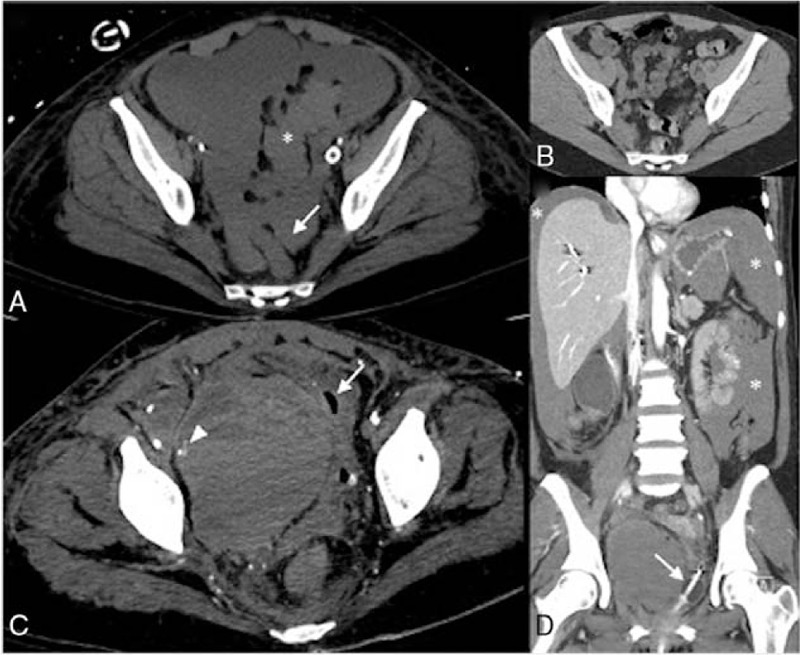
(A) Axial computed tomography (CT) at the level of the sacral promontory shows hemoperitoneum with fluid collection in the pelvis, including the retrouterine space (*arrow*), enveloping the sigmoid colon (*asterisk*). (B) Premorbid axial CT of the patient (obtained for abdominal pain) at identical level as in (A) highlights normal pelvic anatomy by means of comparison. (C) Contrast-enhanced axial CT obtained 15 d after that in (A) shows a large right-sided pelvic retroperitoneal hemorrhage with contrast extravasation (*arrowhead*), posterior compression of the rectum, and leftward displacement of the bladder with intravesical air introduced by catheterization (*arrow*). (D) Coronal reconstruction of CT from (C) shows the pelvic hematoma displacing an inflated Foley catheter (*arrow*) and highlights the large hemoperitoneum (*asterisks*) prior to its evacuation.
